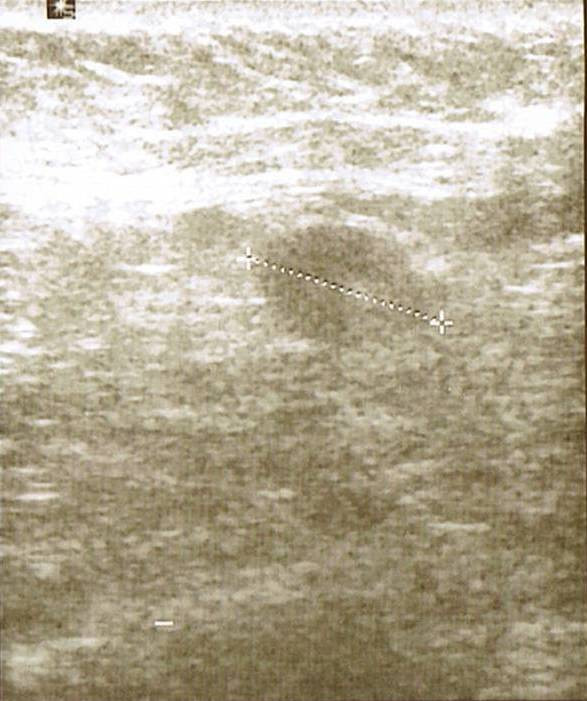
The lymph node is shown on ultrasound with some cortical thickening at its distal pole suggesting focal metastasis.
